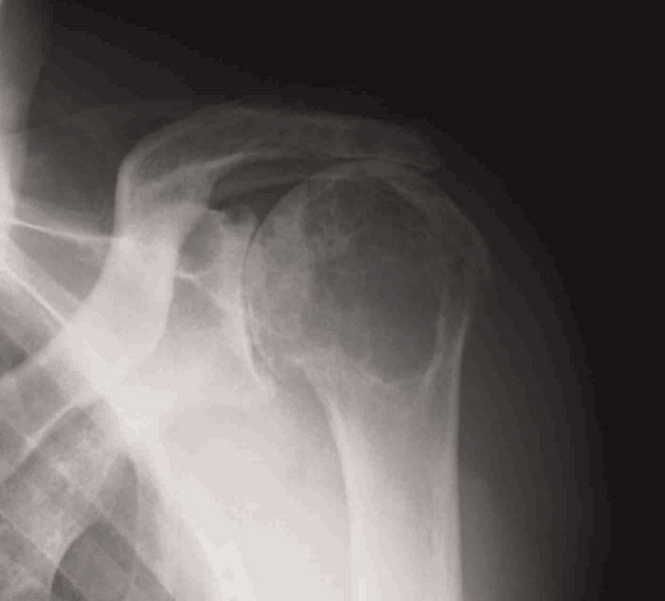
Preoperative shoulder X-ray.
